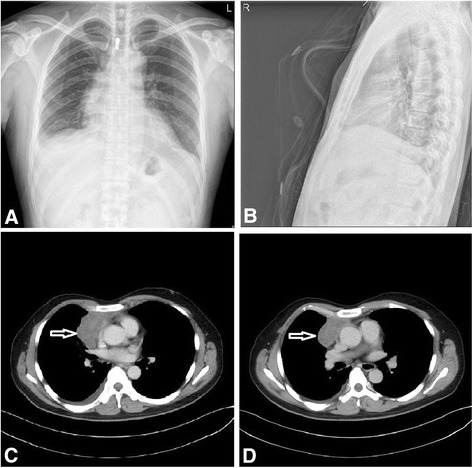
The imaging of the tumor. The X-ray shows a widened mediastinum without displacement (a, b). MRI detected a mass that was approximately 6.4 cm × 4.2 cm in the anterior mediastinum beside the right part of the heart (c, d). The margins between the mass and the pericardium were not clear
